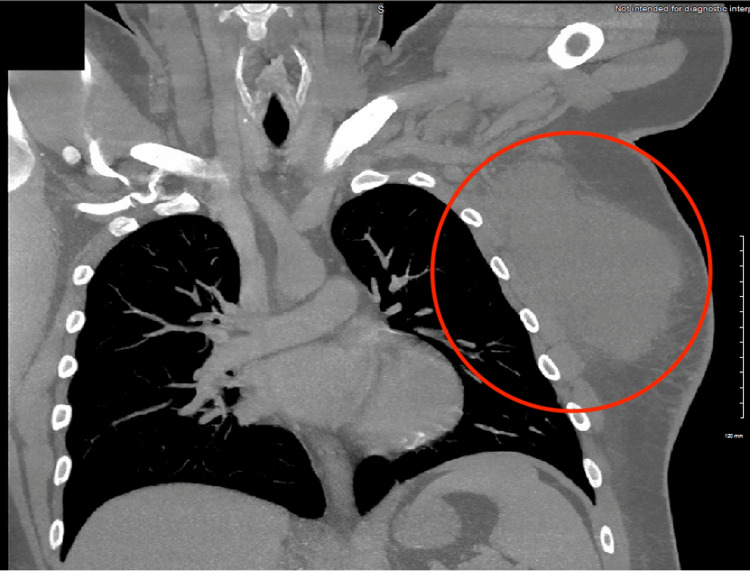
CT (coronal view) of the chest showing a large soft tissue density mass (red circle) involving the left chest wall and axillary region
